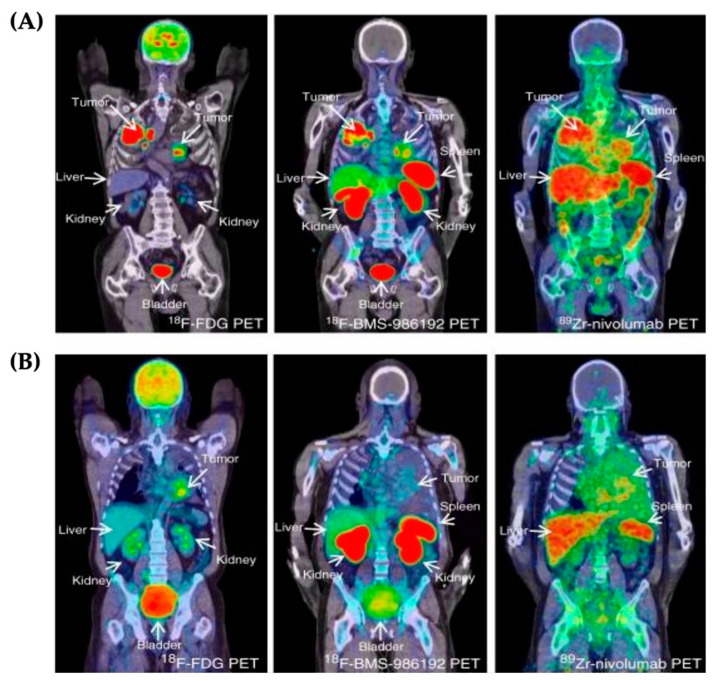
PET/CT images of panel (A,B) are corresponding from two patients, respectively. These images are ^18^F-FDG PET (left), ^18^F-BMS-986192 PET (center) and ^89^Zr-Nivolumab (right).^18^F-FDG PET scan results show malignancies with significant glucose metabolism in both the lungs and the mediastinal lymph nodes. PET with ^18^F-BMS-986192 reveals uneven tracer uptake within and between tumors. ^18^F-FDG PET scans show that the tumor on the left side has a high glucose metabolism. PET with ^18^F-BMS-986192 indicates little tumor tracer uptake. Nivolumab PET with ^89^Zr indicates diverse tracer uptake in the tumor. Adapted from reference [64], licensed under a CC BY 4.0.
